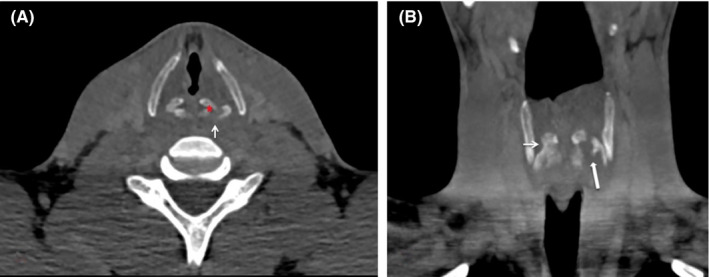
(A) Axial CT demonstrating a fracture of the left posterior cricoid cartilage (arrow). Note the arytenoid cartilage (*) is interposed between the displaced cartilage fragments, (B) coronal maximum intensity projection (MIP) reconstruction demonstrating the widely separated fracture of the left posterior cricoid cartilage (block arrow) resulting in disruption of the cricoarytenoid joint. Note the normal right cricoarytenoid joint (arrow)
